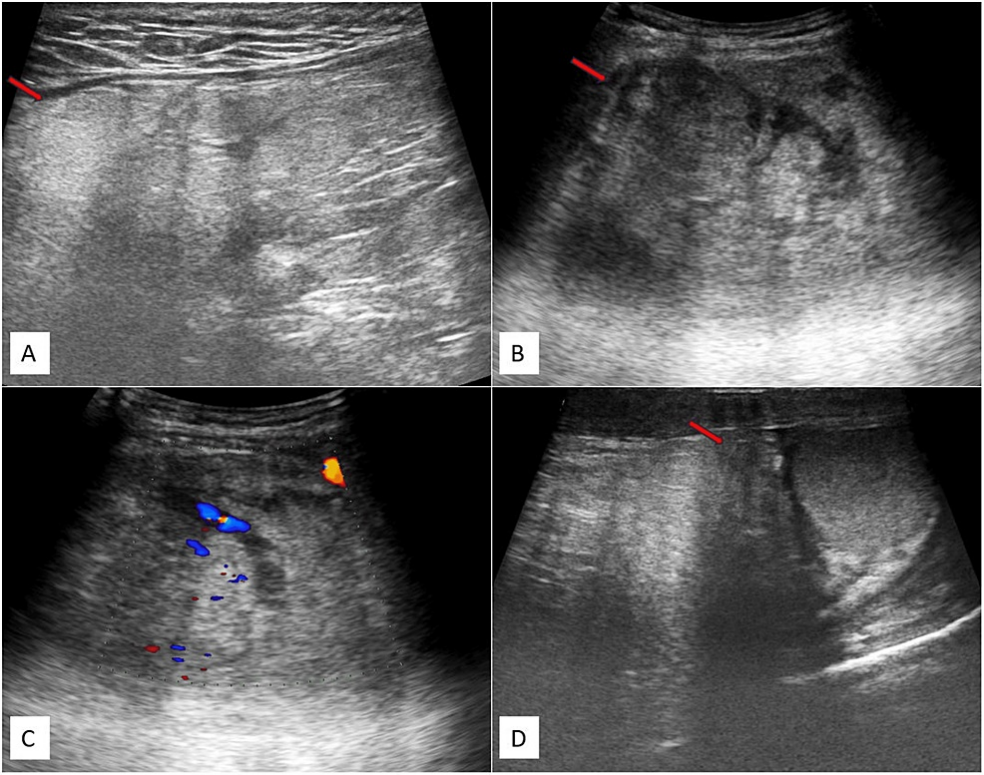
USG images (A-D) of the inguinoscrotal region depict a solid mass with heterogeneous echotexture in the right inguinal region. The mass extends into the right scrotal sac, reaching up to the upper pole of the right testes, and shows internal vascularity. The USG image reveals a solid mass with heterogeneous echotexture in the right inguinal region, where the superior margins could not be well assessed (red arrow) (A), extending into the right scrotal sac (red arrow) (B), displaying mild internal vascularity (C), and reaching up to the upper pole of the right testis (red arrow) (D). USG: ultrasonography
